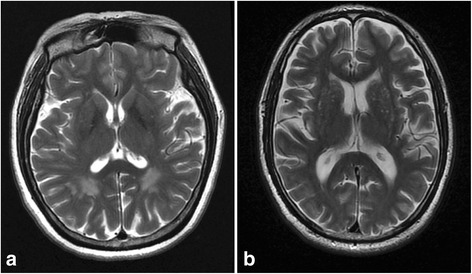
Typical T2-weighted MRI images a control and a OPVHs patient. Two typical T2-weighted MRI images of a control (a) and a OPVHs patient (b). High-signal intensities are observed on the T2-weighted image of the OPVHs patient. In addition, no cerebral infarcts were observed on the T2-weighted images in any subjects
